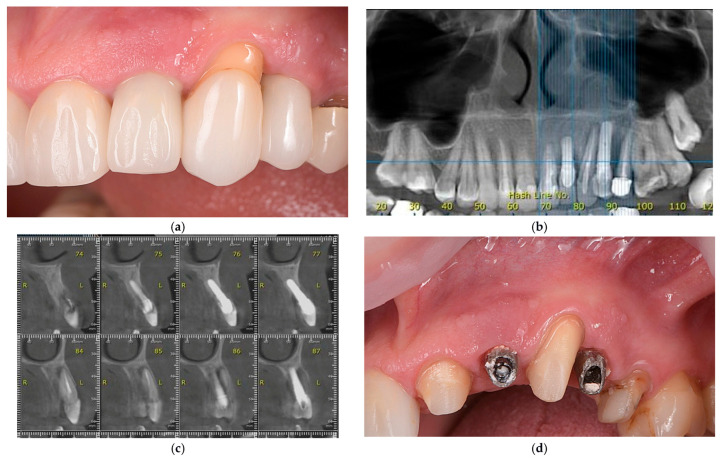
Representative clinical and radiographic images at presentation. (a) Clinical aspect of the implant-supported crowns and adjacent crowns on natural teeth; (b,c) CBCT scan confirming bone loss around implants (The letters “R” and “L” indicate the right and left sides, respectively); (d) Aspect of implant abutments and surrounding soft tissues after crown removal.
